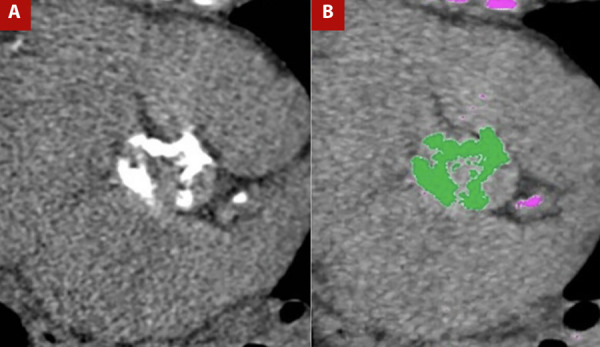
A) Non-contrast cardiac-gated CT in the aortic valve plane for calcium assessment and B) Post-processing for calcium quantification. Marked calcifications are present in the aortic valve leaflets, yielding a calcium score of 2889 Agatston units, highly suggestive of severe aortic valve stenosis.
